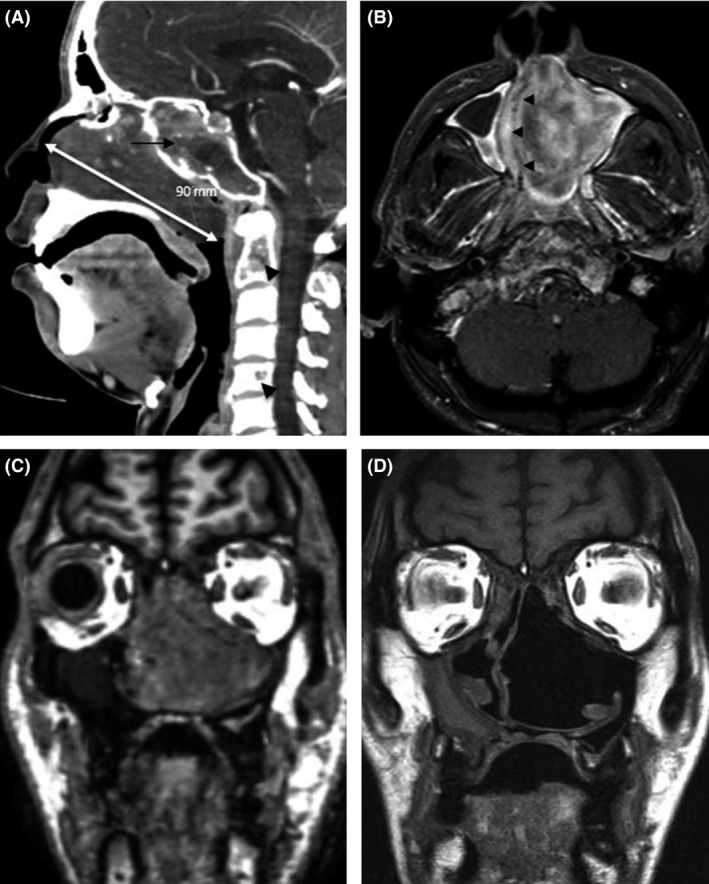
Images of nasopharyngeal mass (A) Preoperative CT. Sagittal view showed a 90 mm heterogeneous mass in the left nasal cavity (white double head arrow) with obstruction of the ethmoidal sinuses (black arrow) and osteolytic lesions in C2 and C5 (black arrowheads). (B) Preoperative postcontrast T1 image MRI study. Axial views showed marked nasal septum deviation (black arrowheads). (C and D) Pre‐ and postoperative MRI study after complete resection of the nasopharyngeal amyloidoma.
